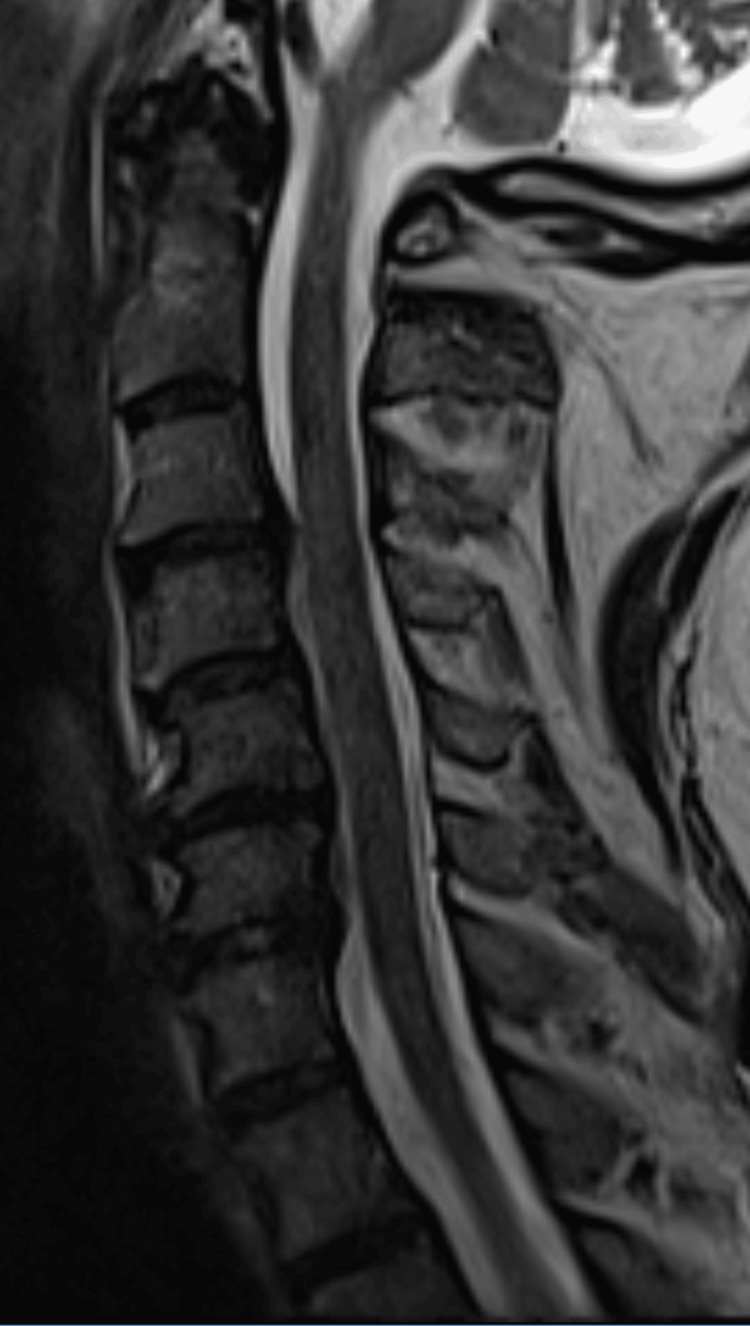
MRI of the cervical spine is unremarkable
